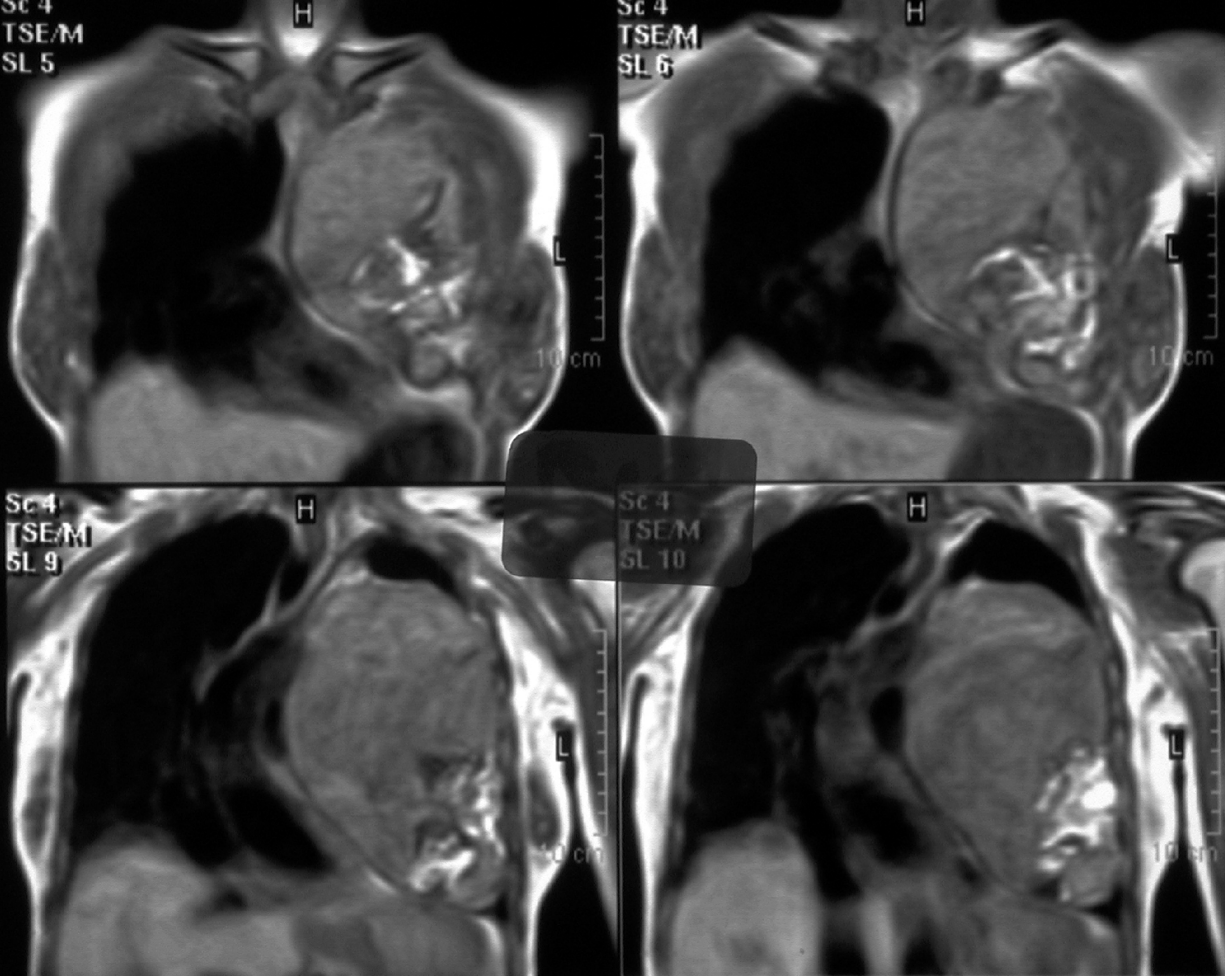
Preoperative MRI showing the teratoma, its anatomic relations to the mediastinum, and its expansion to the left hemithorax.
